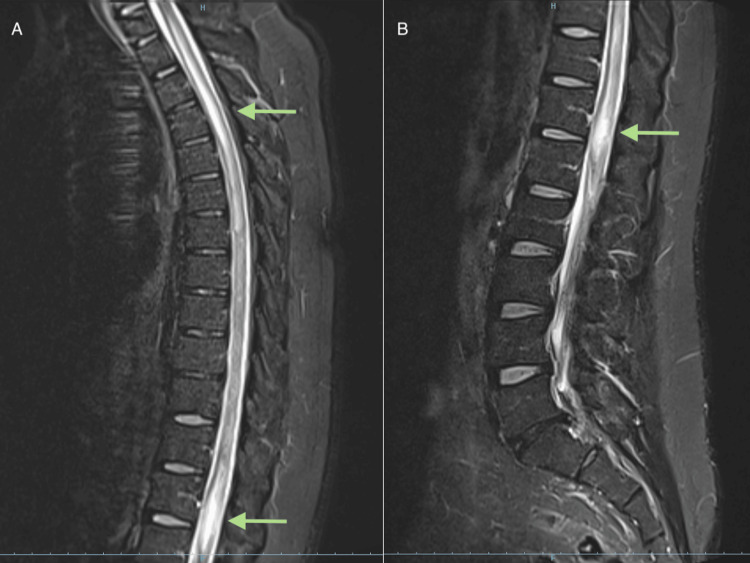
Initial (A) dorsal and (B) lumbar spine MRI images, showing hyperintensities in sagittal short-tau inversion recovery sequence (green arrows) MRI: magnetic resonance imaging
